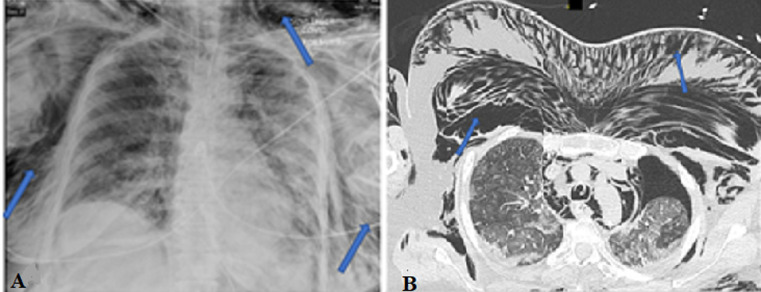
A) the X-ray chest AP view showing significant subcutaneous emphysema; B) the CT scan of chest with the blue arrows showing extensive subcutaneous emphysema
